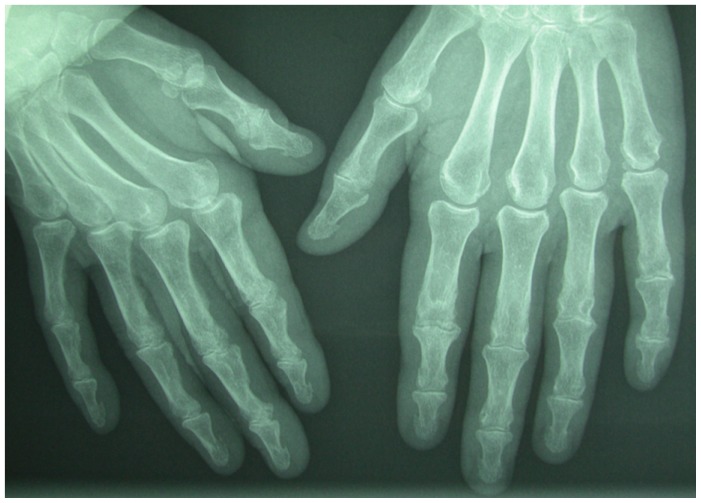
Hand radiograph enhanced by using mammography film (showing subperiostal bone resorption at the inner part of the terminal thumb phalanges symmetrically)
